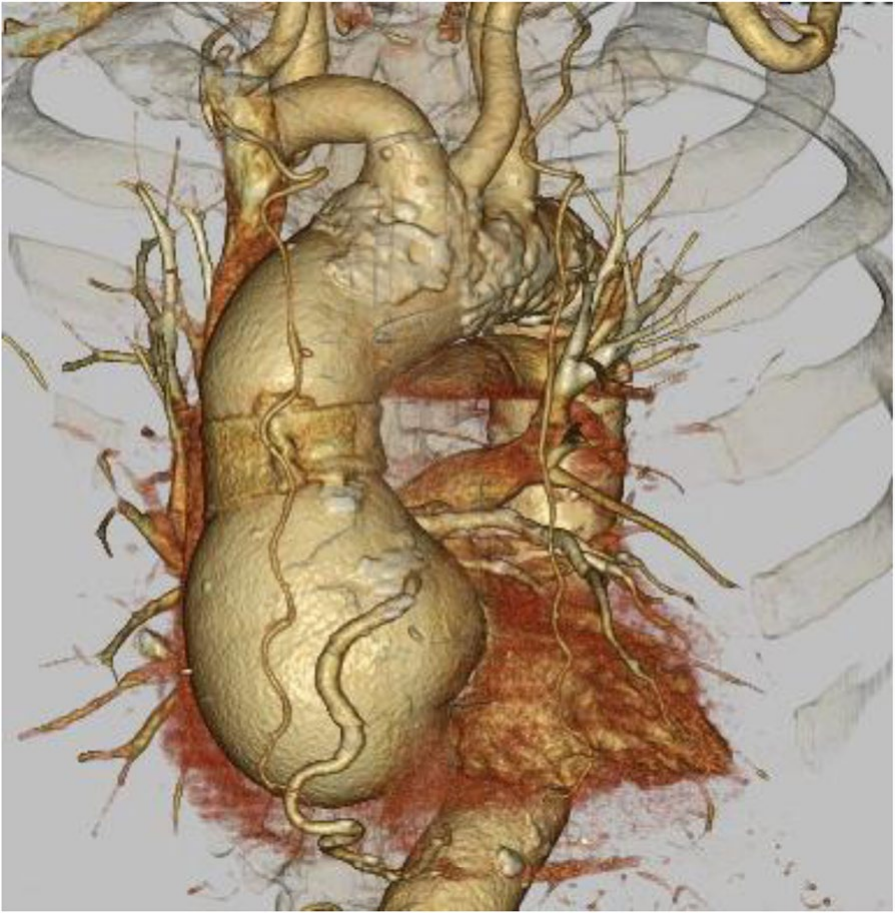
Preoperative three-dimensional computed tomography. The sinus of Valsalva was 62 mm in diameter
